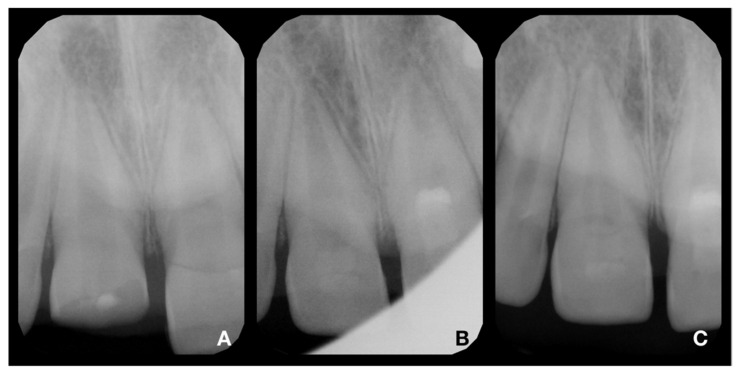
X-rays of tooth 11. (A)—diagnostic X-ray of tooth 11; (B)—postoperative X-ray of tooth 11; (C)—control X-ray at 24-month follow-up. The continuing development of the root and narrowing of the root canal is visible. In addition, the formation of a radiopaque bridge under the calcium silicate cement and the absence of periapical radiolucency are apparent.
